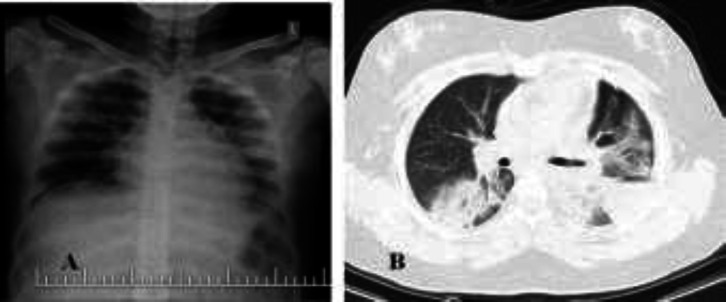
A, B CXR and lung CT findings: ground-glass opacity and consolidation in upper and lower lobes of left lung and right lower lobe
